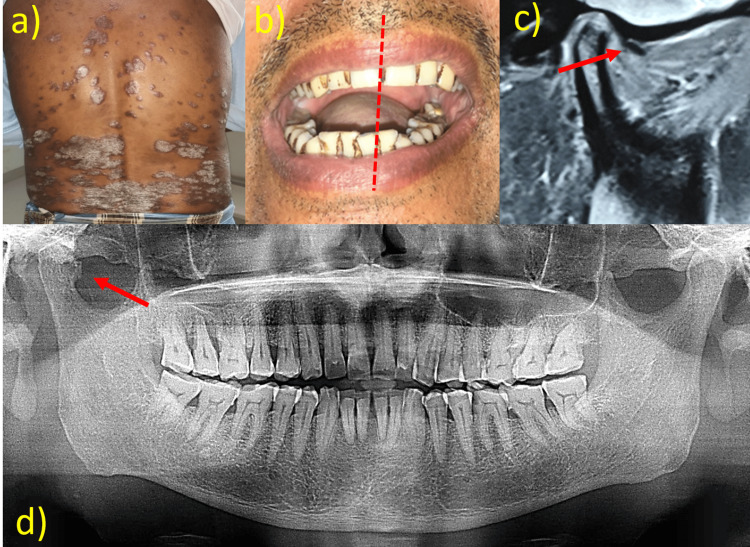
a) Presence of psoriatic patch over the back of the body, b) restricted mouth opening along with deflection on the right side, c) MRI of the right TMJ reveals an anteriorly placed articular disc, d) panoramic radiograph reveals an osteophyte with bony erosion and Ely’s cyst in relation to the right condyle TMJ: temporomandibular joint
